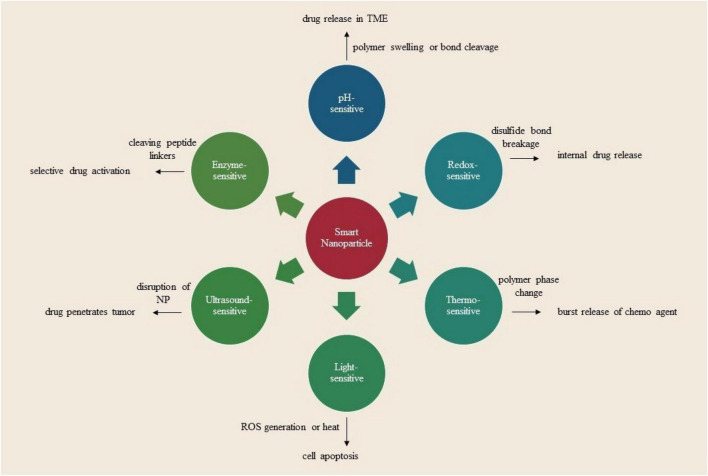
Overview of smart nanocarriers designed to respond to tumor-specific stimuli for on-demand drug release. Various external and internal cues, including acidic pH, high glutathione concentrations, enzymatic activity, elevated temperature, light, and ultrasound, trigger structural changes or cleavage reactions in the nanocarriers, leading to controlled and localized release of therapeutic agents within the tumor microenvironment. These systems aim to improve therapeutic precision while minimizing off-target toxicity.
